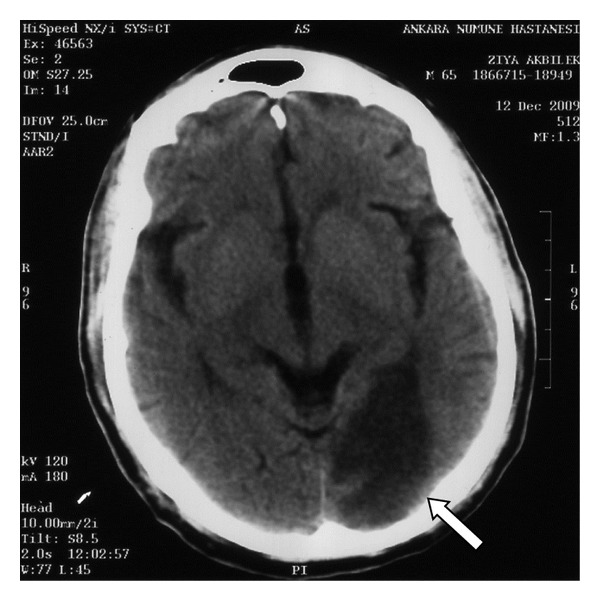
Left occipital infarct area in the CT scan (white arrow).
